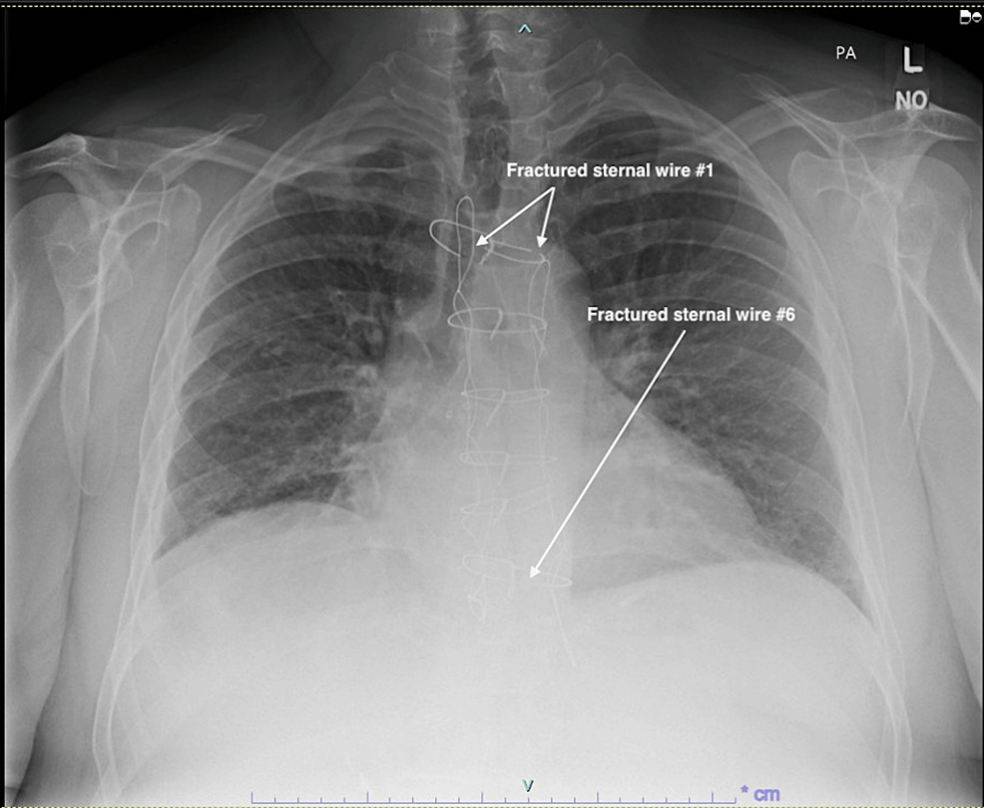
Preoperative chest X-ray image showing the fractured first and sixth sternal wires This postero-anterior (PA) chest X-ray was taken to investigate the symptoms that the patient was experiencing. It clearly shows the first and sixth sternal wires to be fractured. A slight tracheal deviation to the right side is also seen, though this could not be explained and was deemed to be an insignificant finding. The right and left Robicsek wires are seen and appear unremarkable
